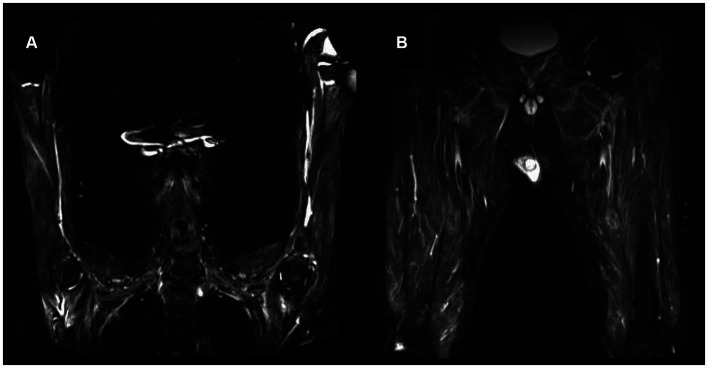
Magnetic resonance imaging (MRI) findings on short-tau inversion recovery (STIR) of proximal upper limb (A) and lower limb (B) in Patient 3. Muscle MRI demonstrated hyperintensity on STIR in muscle and connective tissue surrounding the muscle fasciae of proximal upper and lower limb.
